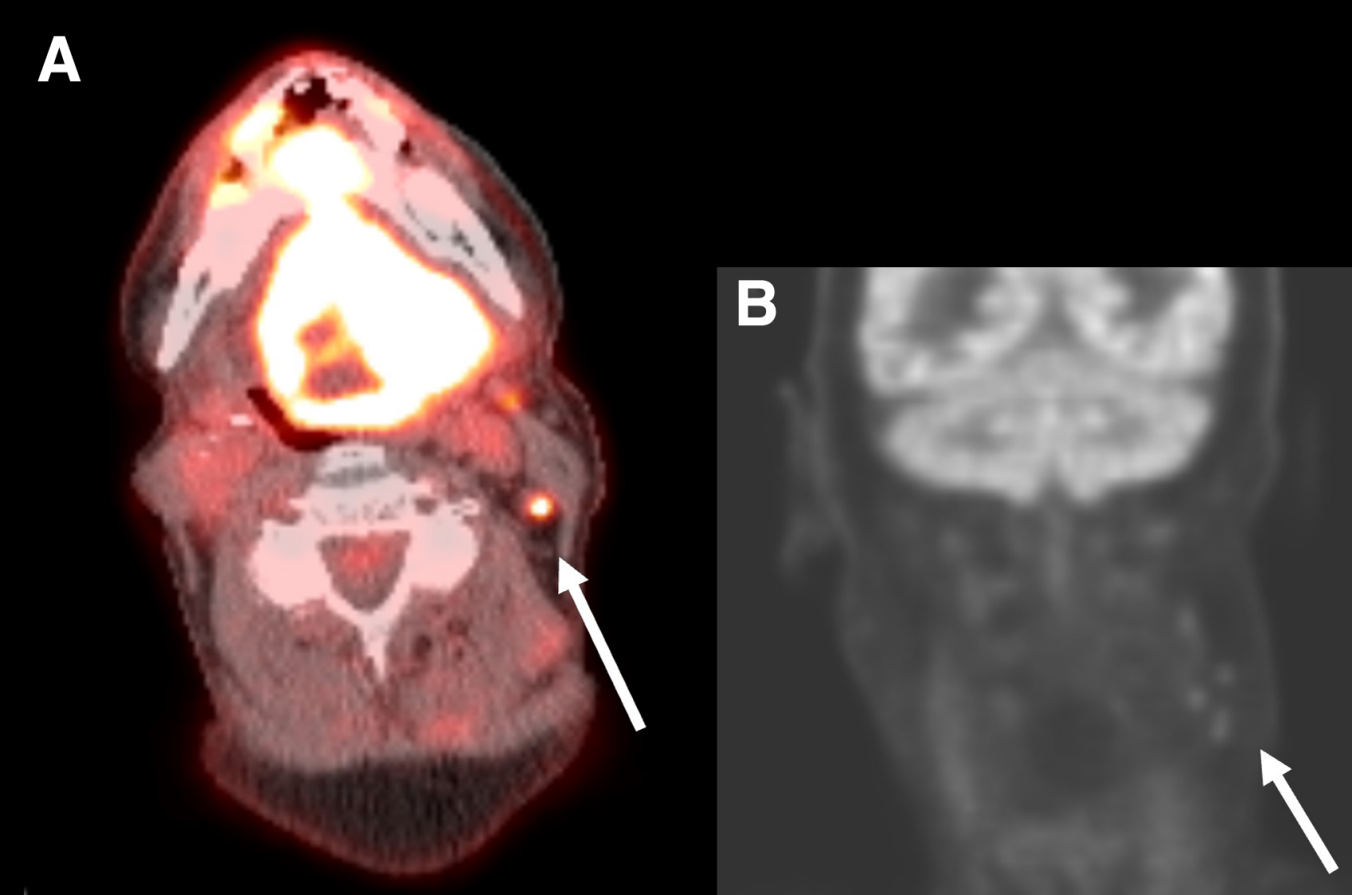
Axial PET/CT fusion of the neck (A) and coronal PET image of the head and neck (B) in a 67-year-old man with a primary SCC involving the entire tongue. Small hypermetabolic lymph nodes are present on the left at levels 2 and 3 (arrows). These were interpreted as suspicious for malignancy by the interpreting radiologist. On surgical pathology no metastatic lymph nodes were present and this patient was classified as a false positive.
